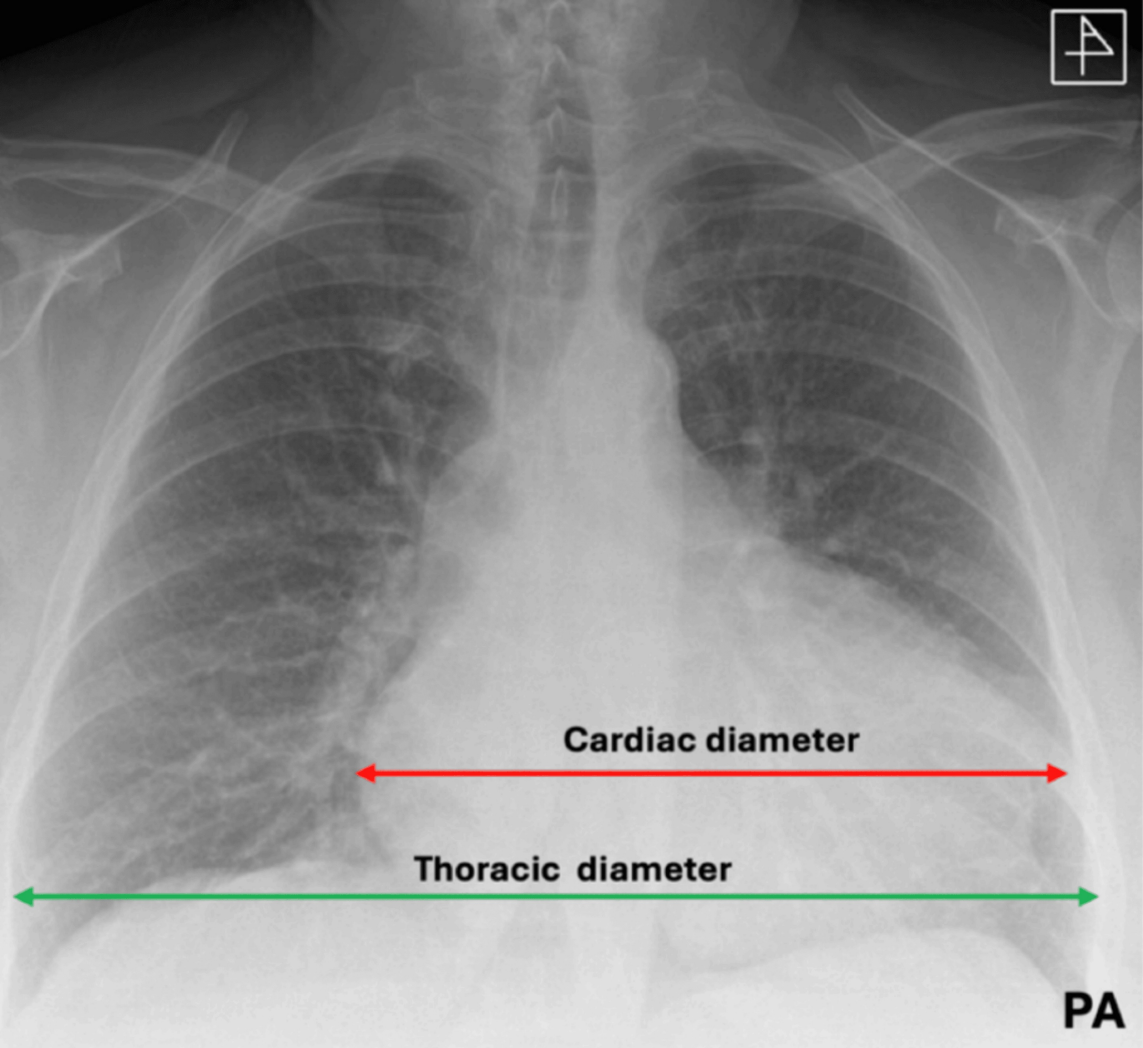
PA chest X-ray film demonstrating enlargement of the cardiac shadow. Cardiac diameter (red arrow) is more than 50% of the thoracic diameter (green arrow) This image has not been published elsewhere. PA: posterior-anterior
